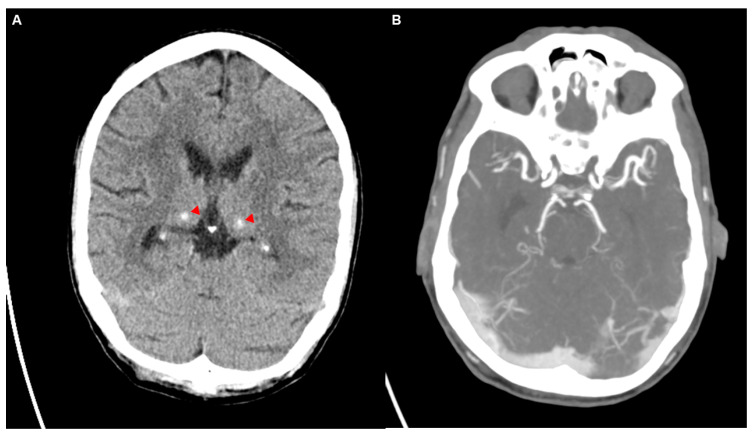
The initial non-contrast CT scan of the head (A) revealed cerebrospinal fluid spaces that were age-appropriate, with no evidence of mass effect or intracranial hemorrhage. There were no signs of acute ischemic changes. Hyperdensities observed bilaterally in the thalami were consistent with calcifications, which were newly noted compared to prior imaging from 2022 (two years before). Additionally, defects that were previously observed in the right temporal lobe on imaging two years earlier were noted again, accompanied by ex vacuo dilation of the right temporal horn of the lateral ventricle. The calcifications of the basal ganglia, although newly observed, were considered incidental and had no clinical significance, consistent with previous reports of age-related or physiological calcifications in the basal ganglia and thalamus [4]. A CT angiography (CTA) (B) of the extra- and intracranial vessels in a mixed arterial and venous phase. There was a calcified plaque in the left vertebral artery, besides that the CTA showed normal arterial and venous structures without any evidence of vascular malformations, stenoses, or aneurysms. There are no signs of thrombosis or other vascular abnormalities, with intact and well-defined vessels throughout the visible cerebral vasculature.
